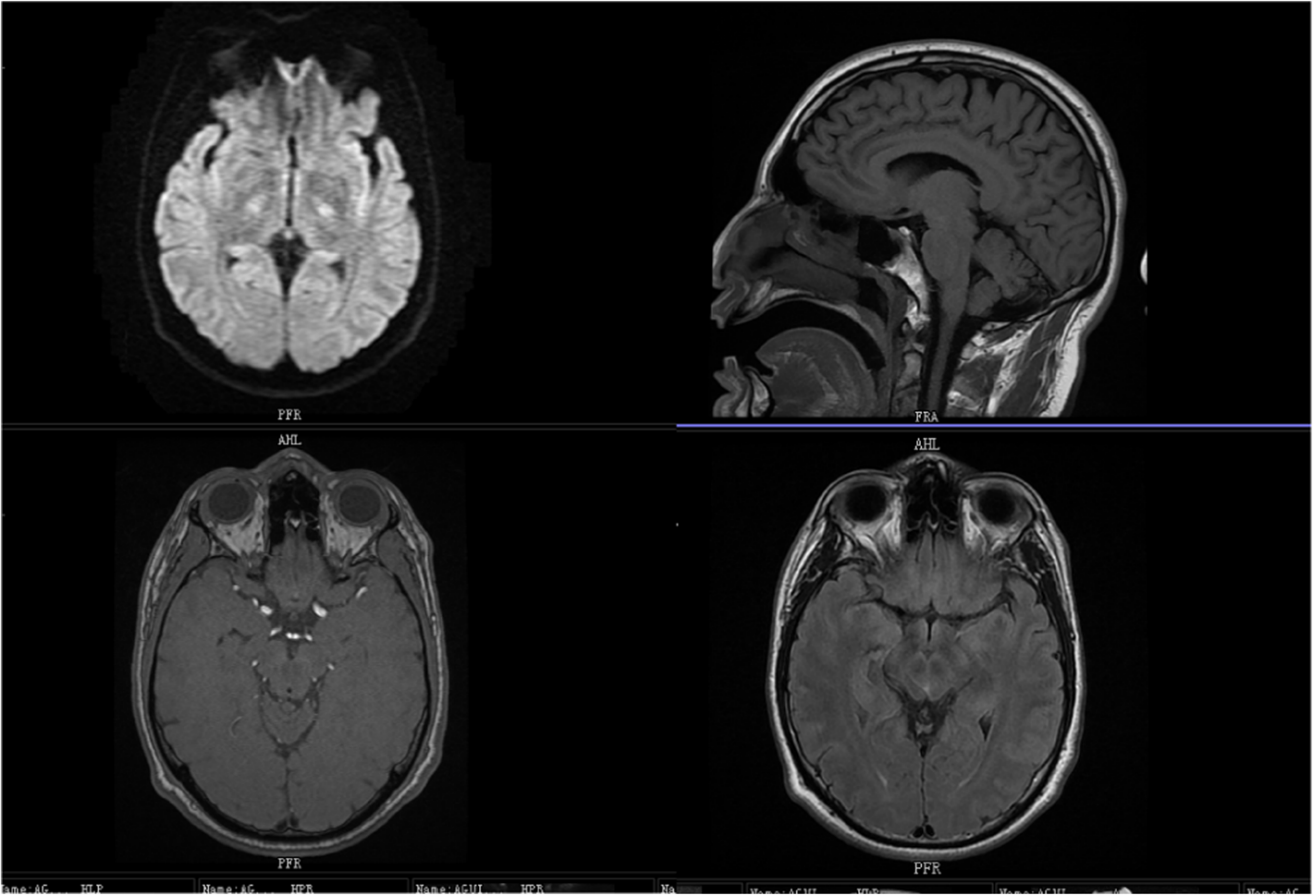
Brain MRI showing no signs of stroke or encephalitis
